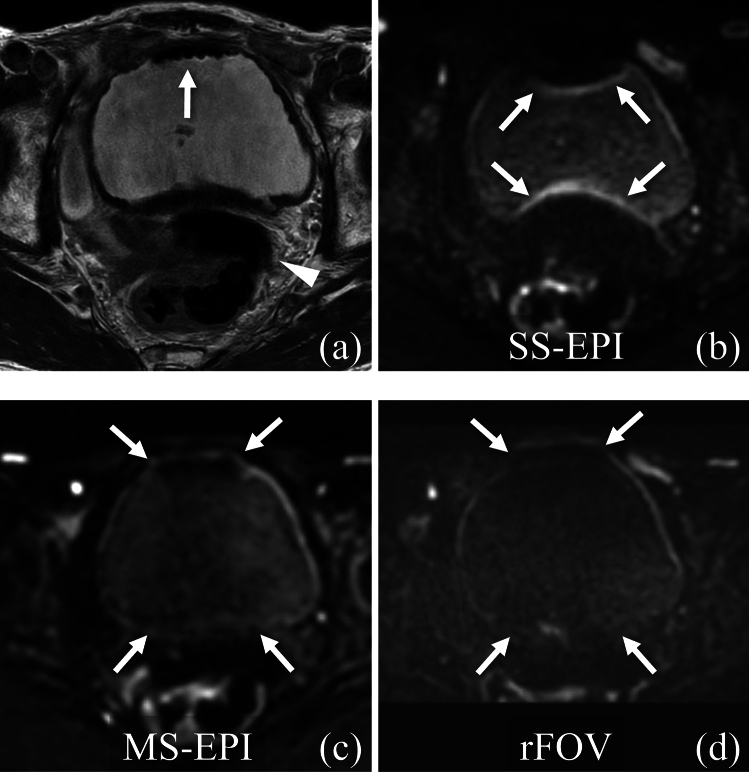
Qualitative evaluation of anatomical distortion. a T2-weighted image shows the presence of gas at the ventral side of the bladder (white arrow) and in the rectum (white arrowhead). b Single-shot echo planar imaging diffusion weighted imaging (SS-EPI DWI). There is severe anatomical distortion (white arrows) due to gas in the bladder and the rectum. c Multi-shot echo planar imaging diffusion weighted imaging (MS-EPI DWI). Although mild anatomic distortion (white arrows) is seen in the anterior and posterior bladder walls, it is markedly improved compared to SS-EPI DWI. d Reduced field-of-view diffusion weighted imaging (rFOV DWI). Although moderate anatomic distortion (white arrows) is seen in the anterior and posterior bladder walls, it is an improvement over SS-EPI DWI
